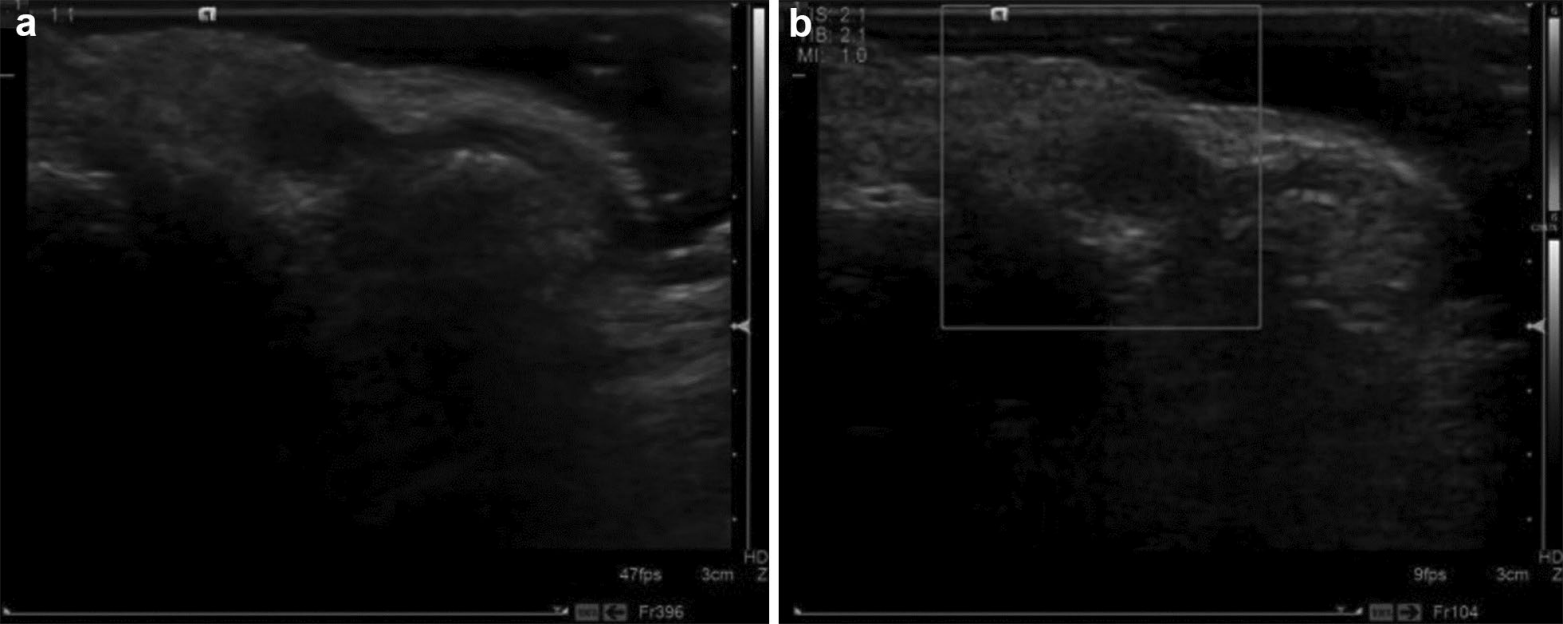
Ultrasound images of female patient (45 years old). a Two-dimensional ultrasound showed hypoechoic in the inside subcutaneous of the second toenail root of the left foot with clear border; b CDFI showed no intratumoral blood flow. The *Figure* indicated that the node was located in subcutaneous tissue adjacent to root of hyponychium
